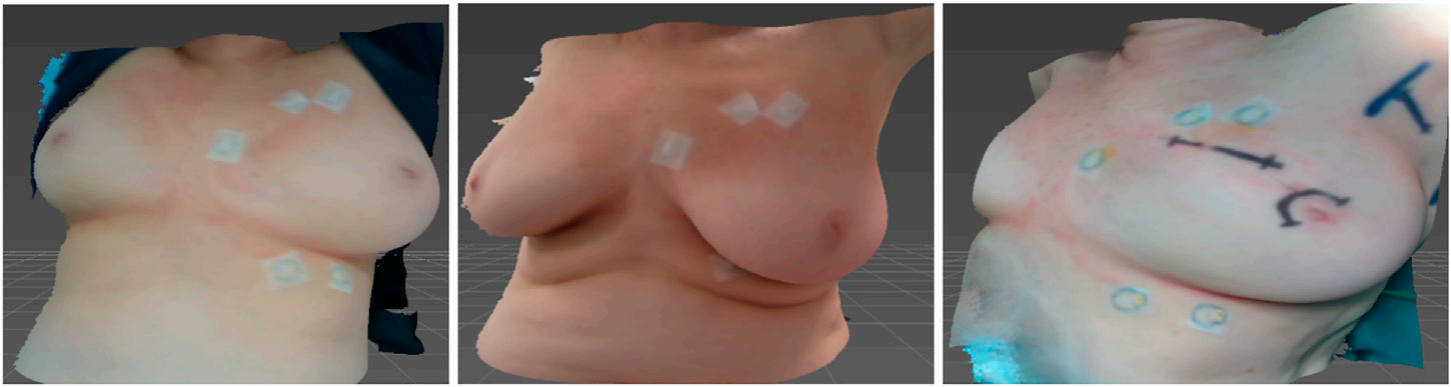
3D surface scans for the same patient in different positions. From left to right: supine position with arms lifted, which corresponds to the regular CT scanning position; sitting position, which we use for parameter estimation; surgical position, used for validation.
